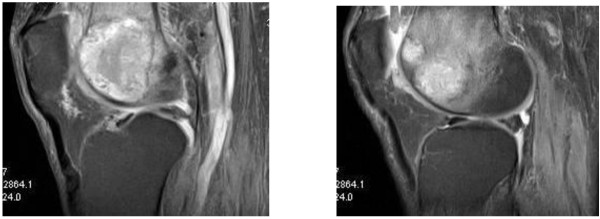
Magnetic resonance image showing knee joint and synovial metastasis. In short TI reversion proton density (STIR PD) sequences; sagittal plane images, there is a lesion in the femur medial condyle epiphyseal-metaphyseal line which has a peripheral hyperintense signal and shows edematous changes in the near bone. In the same sequences, there is an increase of synovial fluid in the suprapatellar pouch and at this location there is another poor hyperintense lesion (bone and synovial metastasis).
